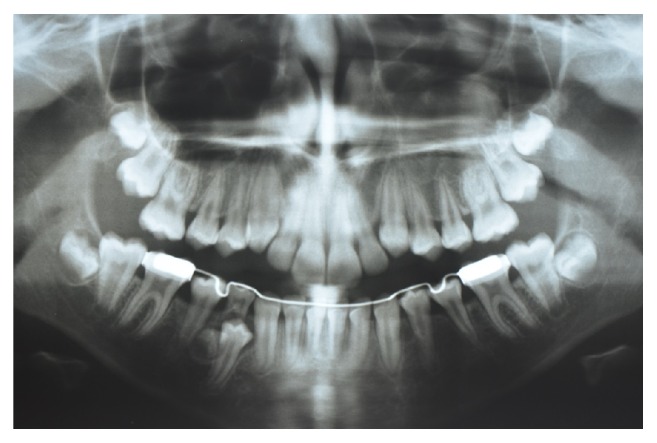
Panoramic radiograph of the patient aged 10 years 5 months showing early formation of a supernumerary tooth in the right mandibular premolar region.
